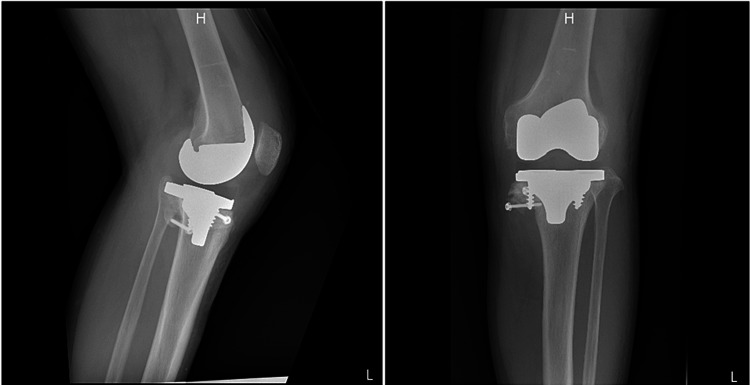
Postoperative radiographs of the left knee. Lateral (left) and anteroposterior (right) views show the restored alignment of the left knee after total knee arthroplasty.
